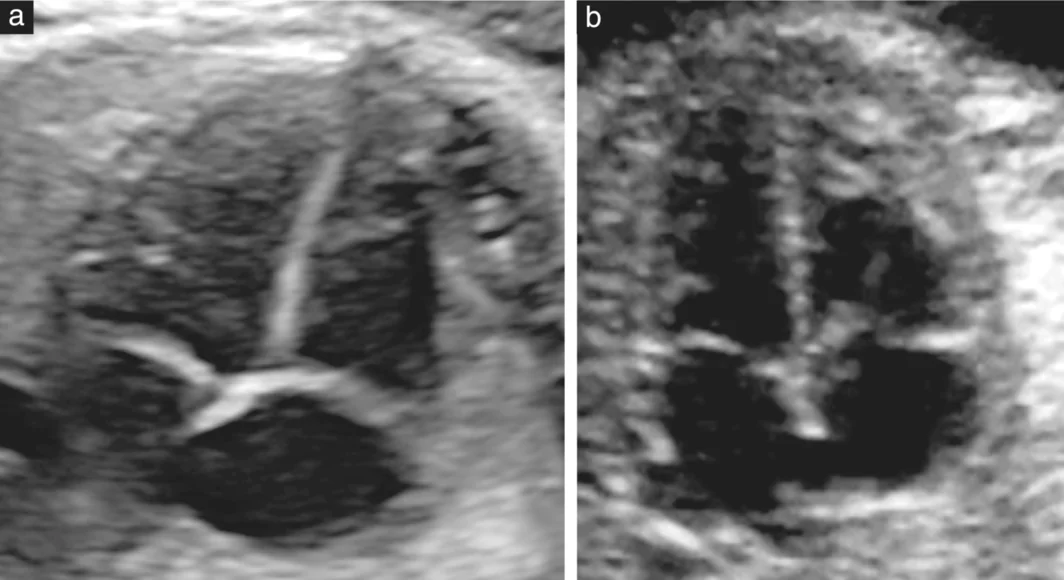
Cardiac morphology in 29 + 6‐week fetus exposed to threatened preterm labor (a) and normal control fetus at 30 + 5 weeks' gestation (b): four‐chamber views obtained by transabdominal two‐dimensional grayscale ultrasound imaging in axial plane, showing more spherical cardiac shape in fetus with threatened preterm labor.
